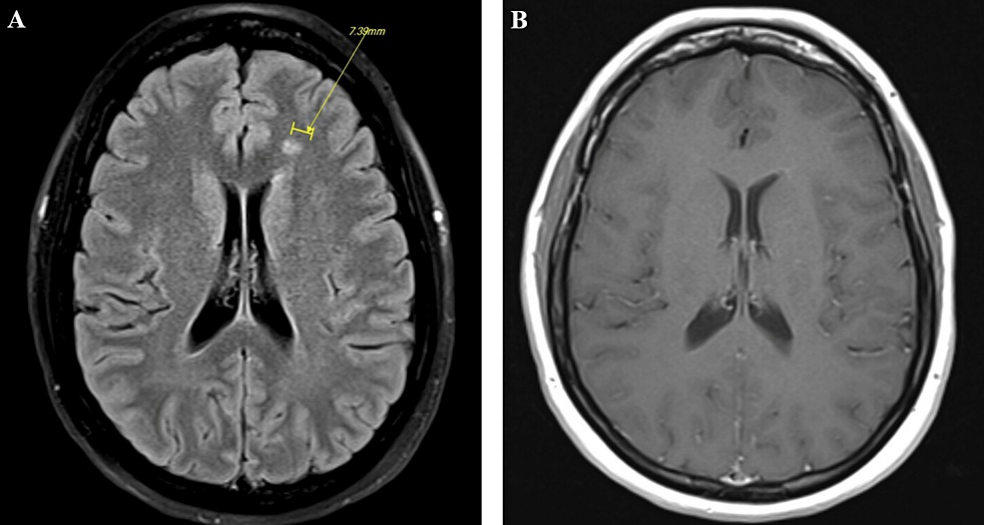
MRI of the brain with and without contrast. A) MRI of brain T2 fluid-attenuated inversion recovery (FLAIR) axial cut; B) MRI of brain T1 post-contrast axial cut Diffuse abnormal hyperintensity appreciated specifically a small T2 hyperintense foci in the deep white matter of the left frontal lobe, superior to the left frontal horn with no contrast enhancement seen on post-contrast imaging.
